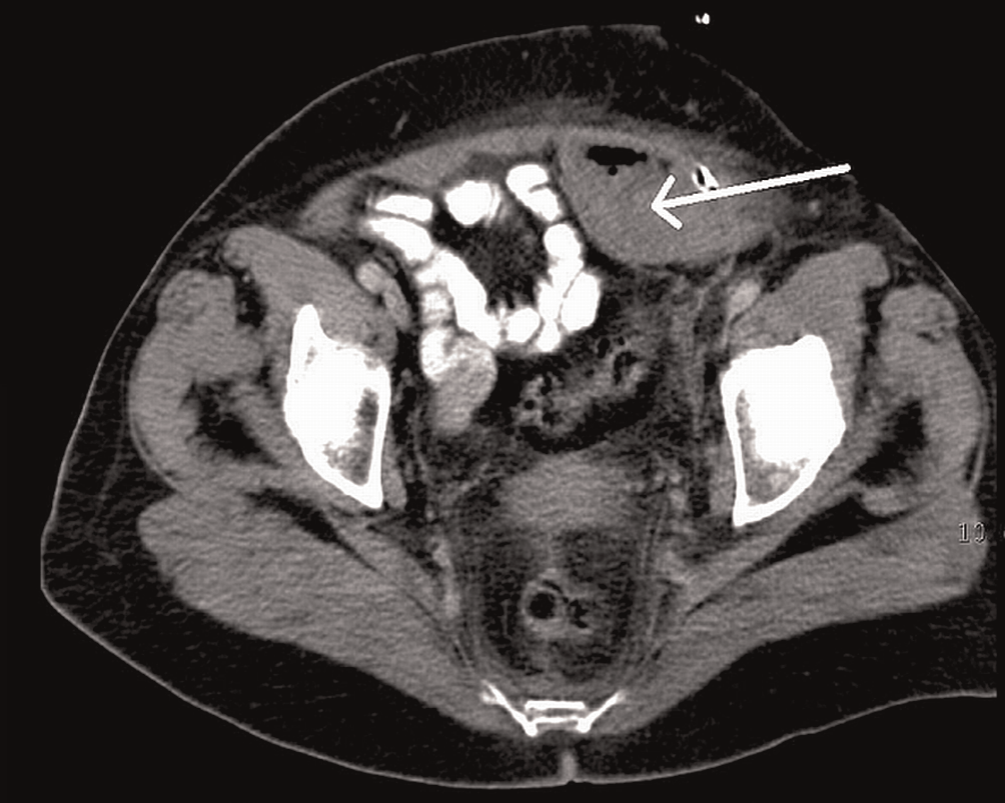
Computed tomography scan showing haematoma in the left rectus sheath (arrow).
